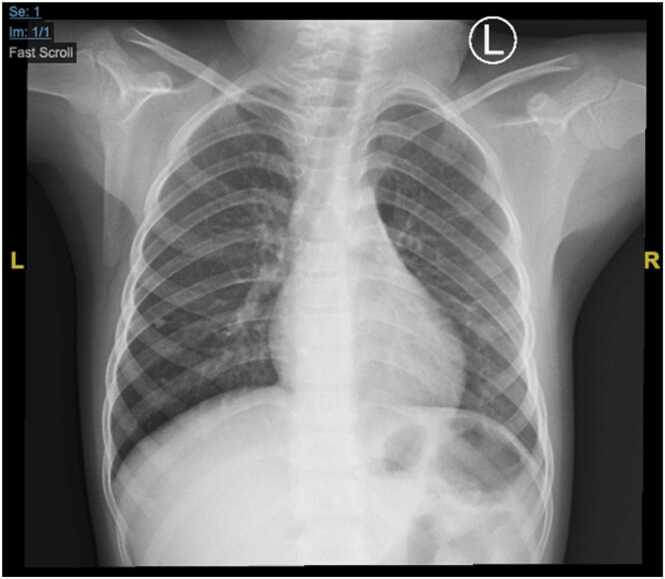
Chest X-Ray revealed normal appearance.
